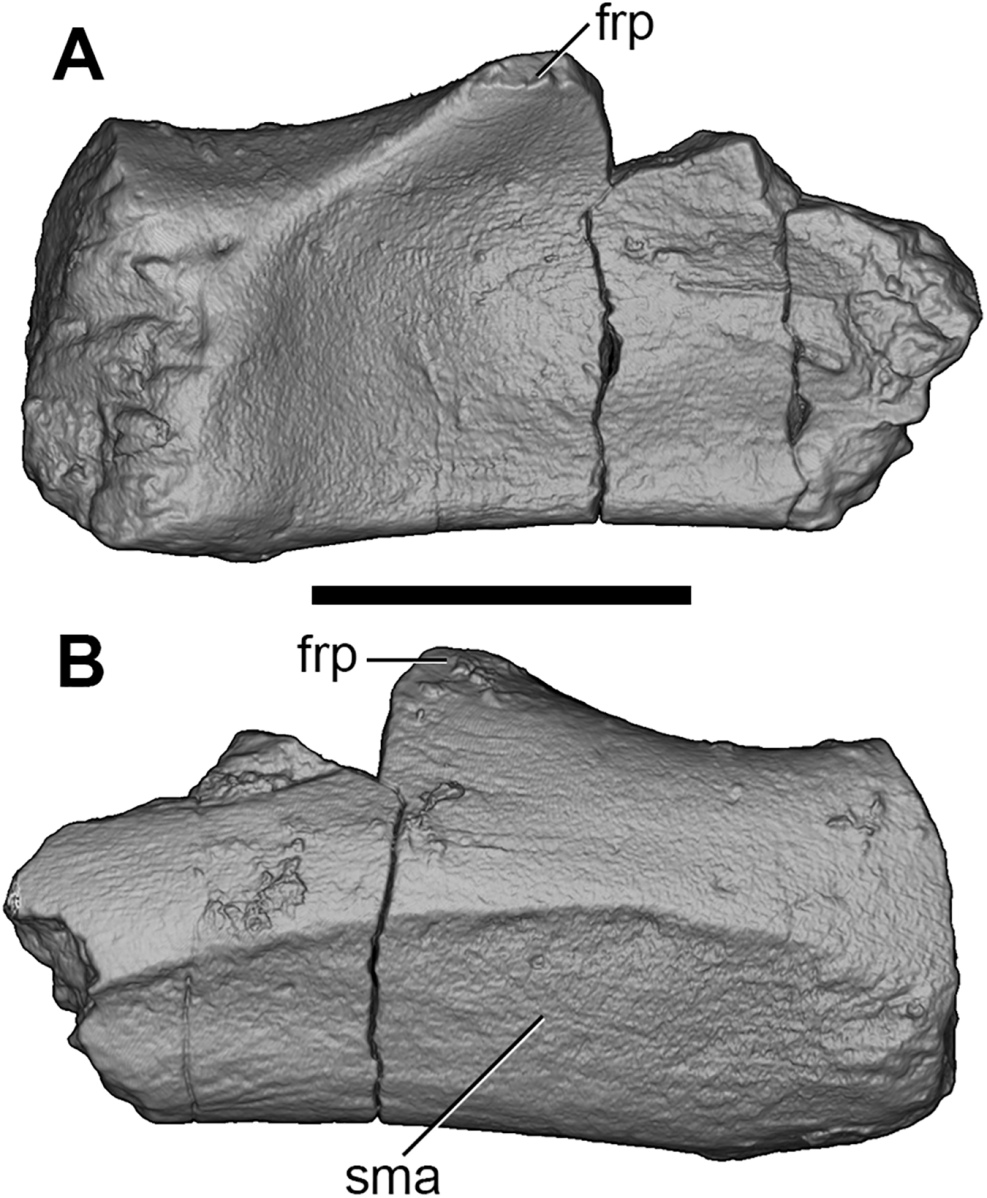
Volume-rendered CT images of right jugal of holotype of *Anatoliadelphys maasae* (AÜJM 2002–25). A, medial view; B, lateral view. Abbreviations: frp, frontal process of the jugal; sma, sulcus for masseter muscle. Scale bar = 1 cm.
